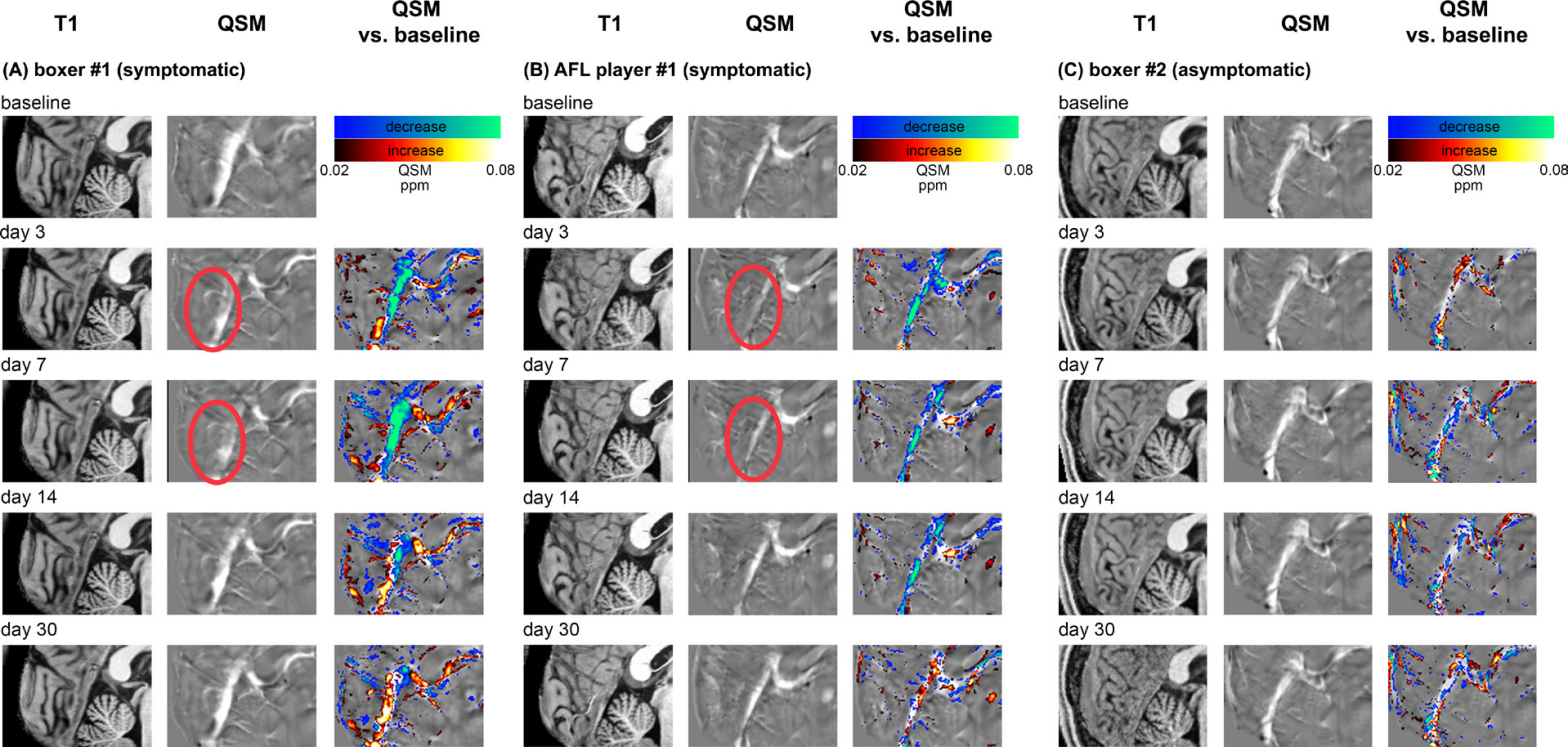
The evolution of the T1-weighted structural images, QSM and the change in the susceptibility maps in the three participants who returned for follow-up scans, all relative to individual baseline values. QSM, quantitative susceptibility maps.
